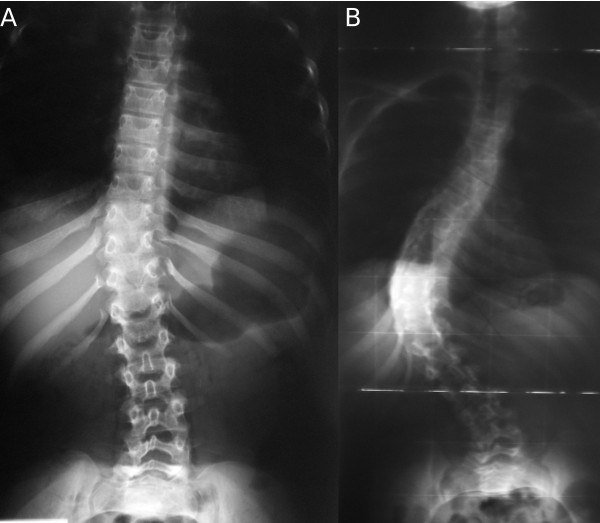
(A, B), Case 1: Spine radiographs at the age of 1 year and 14 years, respectively.
